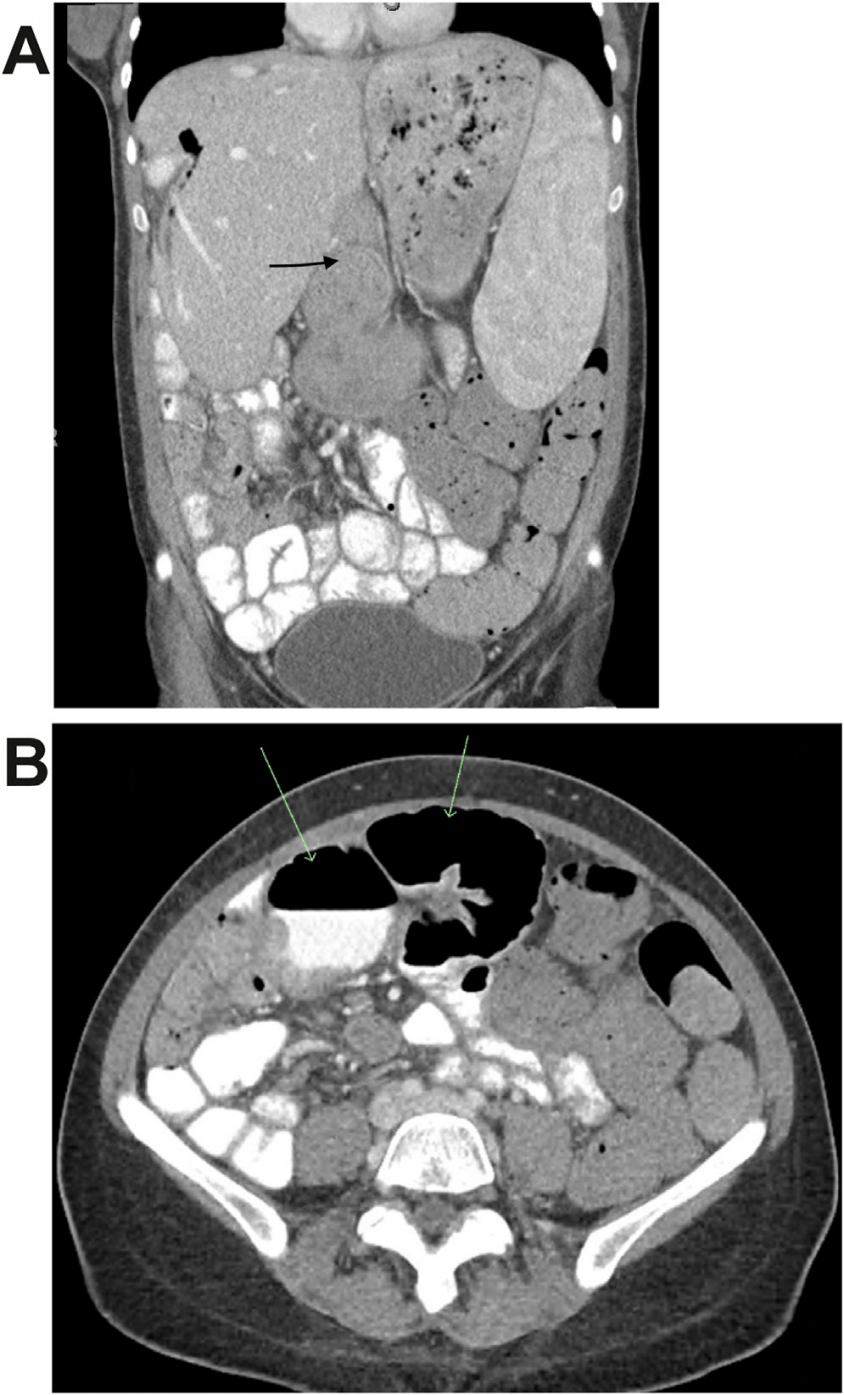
(A) Coronal view CT showing a dilated afferent jejunal segment (B), transverse view CT with green arrows showing dilated afferent jejunal segment. CT, computed tomography.
